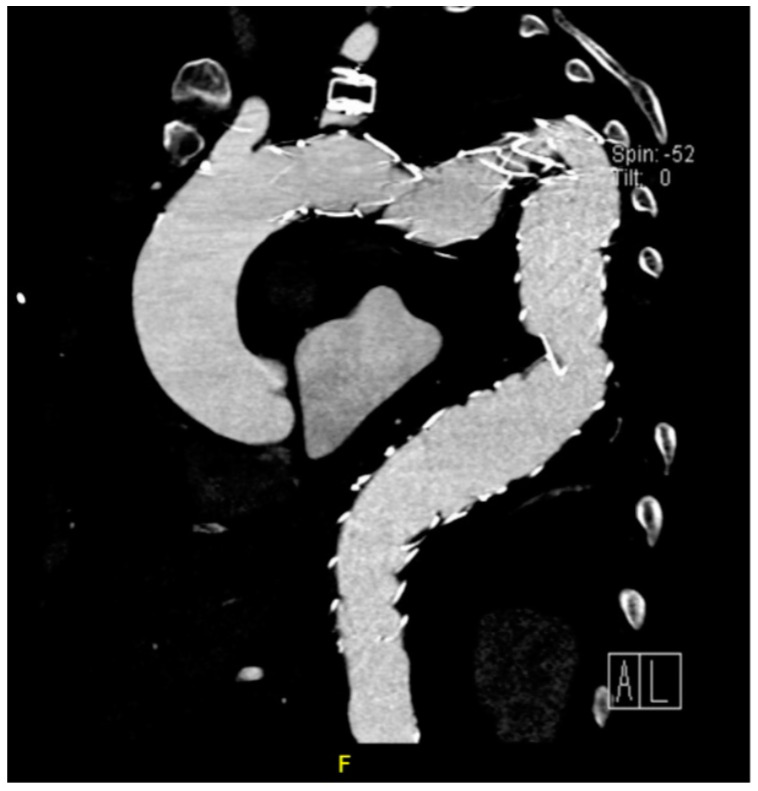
Postoperative CT scan after TEVAR.
